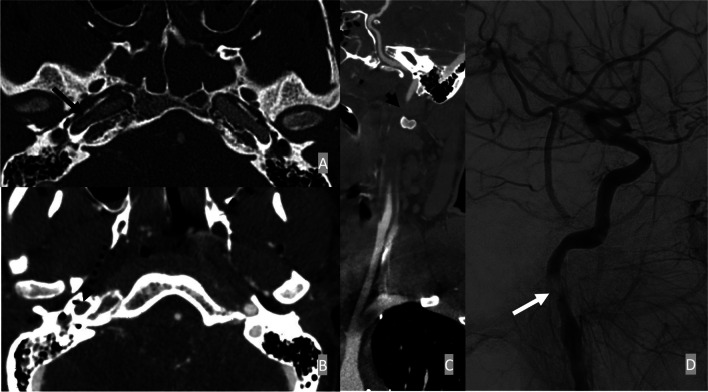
Petrous ICA dissection. A 19-year-old male presents after MVC with left parietal open depressed skull fracture, nondisplaced right temporal bone fracture, and diffuse SAH. The initial CTA demonstrated vessel irregularity of the proximal right petrous ICA (A). There was an absence of contrast opacification from the proximal cervical ICA to the proximal petrous ICA (B and C). Right lateral ICA injection of DSA (D) demonstrated Biffl Grade II flow-limiting dissection of the right petrous ICA
